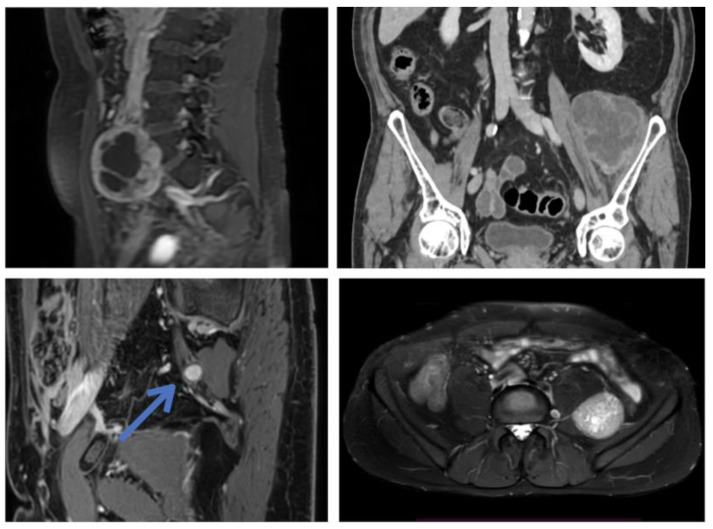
Preoperative MRI images of patients 9 (top left), 15 (top right), 8 (bottom left), and 13 (bottom right) showing the heterogeneous radiological features of retroperitoneal PNST.
